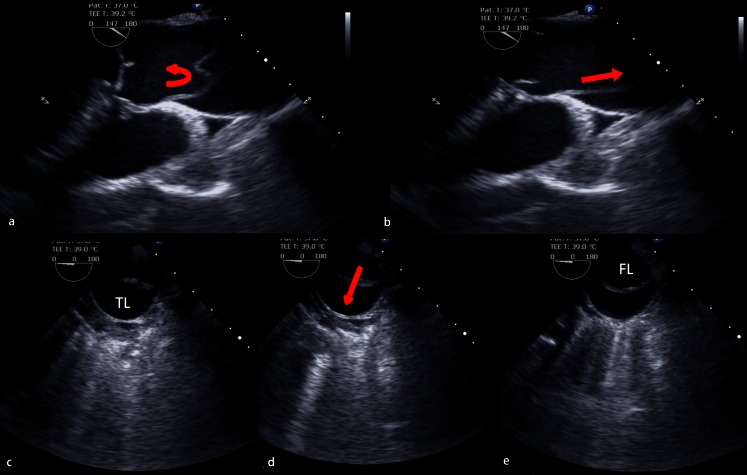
Transesophageal echocardiography (*TEE*) imaging demonstrating the infolding and unwinding (*arrows*) of the intimal flap at the level of the ascending aorta (a, b), and fluctuation of the intimal flap at the level of the descending aorta during the cardiac cycle (c, d, e) (*TL* true lumen, *FL* false lumen)
